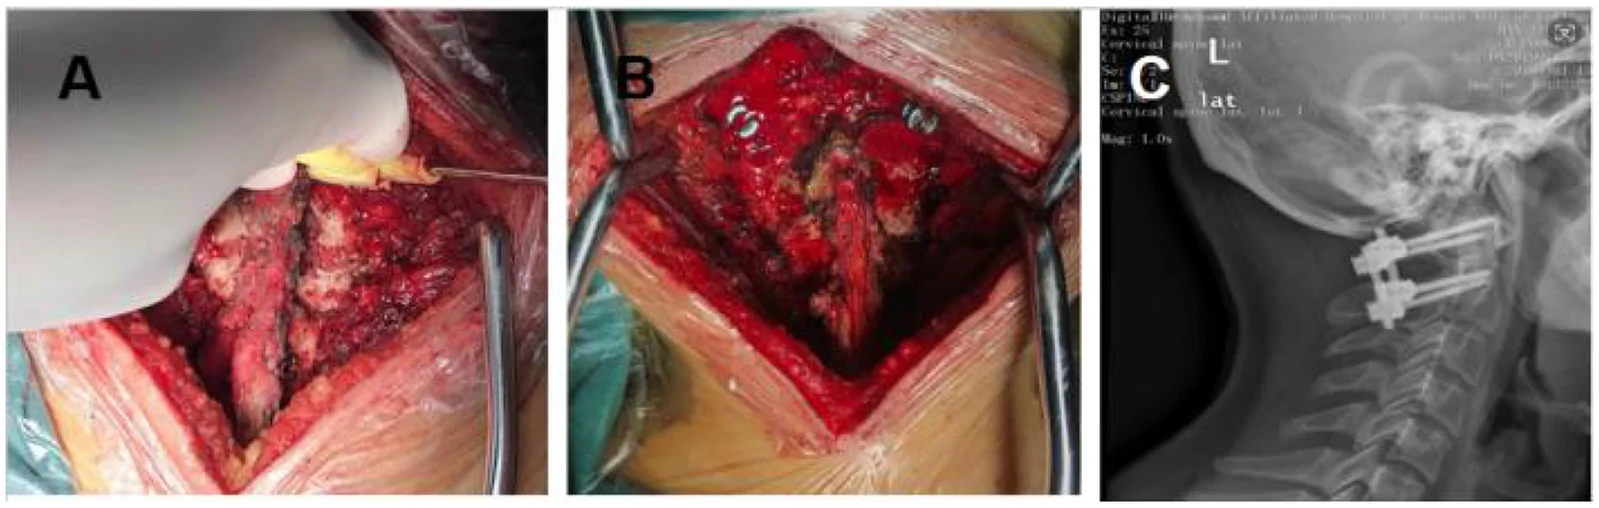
Intraoperative use of the guide for screw placement. (A) Drilling after the guide is fitted to the bone surface during surgery; (B) Intraoperative screw placement; (C) Postoperative X-ray review.
